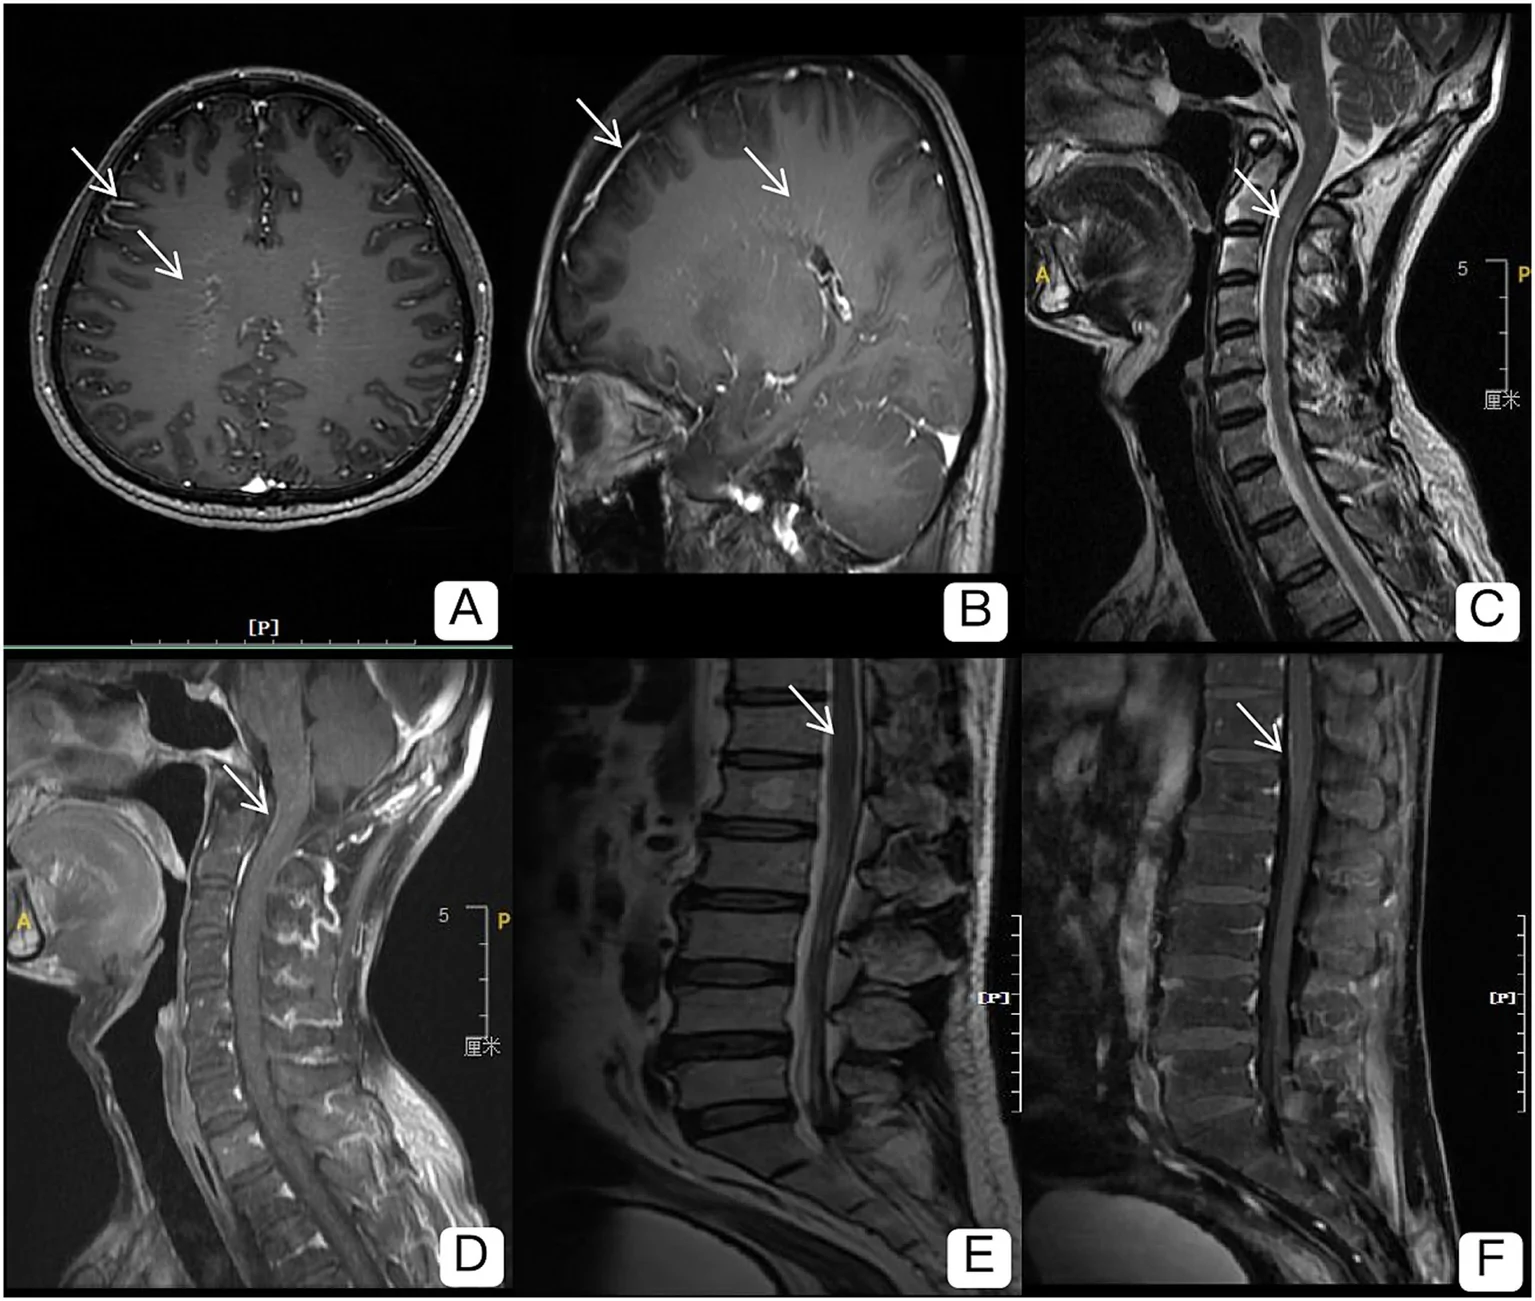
Representative brain and spinal MRI findings in patients with autoimmune GFAP astrocytopathy and CSF EBV positivity. Contrast-enhanced axial brain MRI shows characteristic periventricular radial linear enhancement (A,B). Sagittal cervical spinal cord t2-weighted and contrast-enhanced t1-weighted images demonstrate intramedullary signal abnormalities and leptomeningeal enhancement (C,D). Sagittal lumbar spinal MRI demonstrates spinal leptomeningeal and parenchymal inflammatory abnormalities (E,F). CSF, cerebrospinal fluid; EBV, Epstein–Barr virus; GFAP, glial fibrillary acidic protein; MRI, magnetic resonance imaging.
